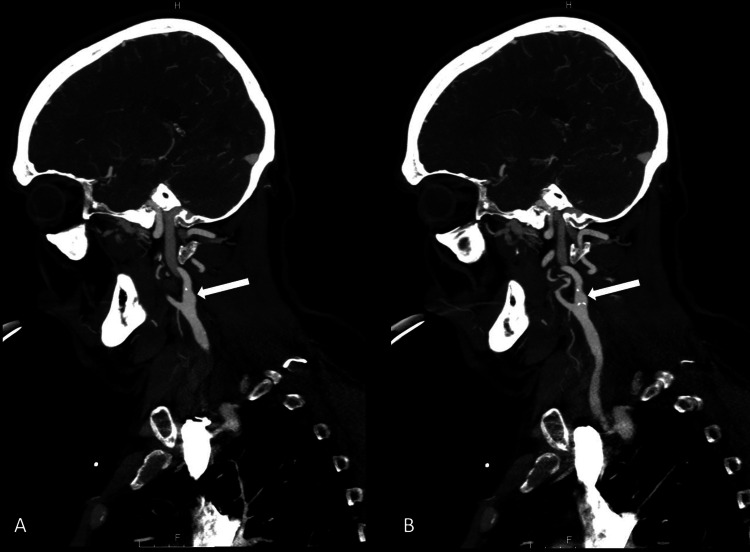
CTA Head and Neck: two sequential images A & B, using 5mm slices, demonstrates widely patent left ICA (arrow), post CEA CTA: computed tomography angiography; ICA: internal carotid artery; CEA: carotid endarterectomy
